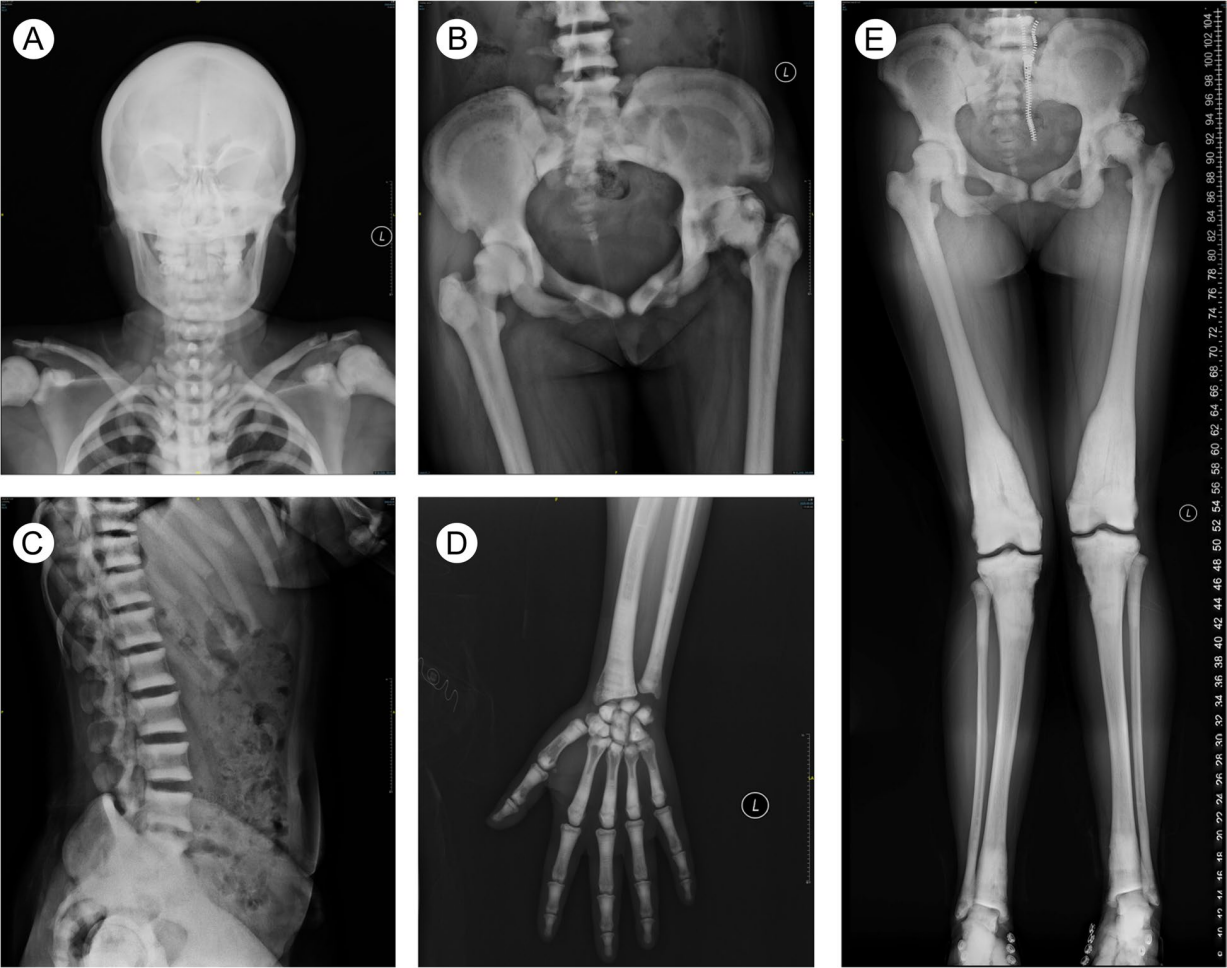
X-ray evaluation of an autosomal dominant osteopetrosis type 2 (ADO-2) phenotype. A AP skull radiograph showing generalized increase in bone density. B Sclerosis in the iliac wings, acetabuli and femoral heads. C Lateral thoracic and lumbar spine radiograph with dense sclerotic bands at the vertebral body endplates (‘sandwich vertebrae’). D AP phalanges radiograph with endobone (‘bone-in-bone’) appearance. E A full-length standing anteroposterior radiograph of both lower limbs showing diffused bone sclerosis, ‘Erlenmeyer flask’ deformity, and ‘bone-in-bone’ appearance. AP = anterior–posterior
